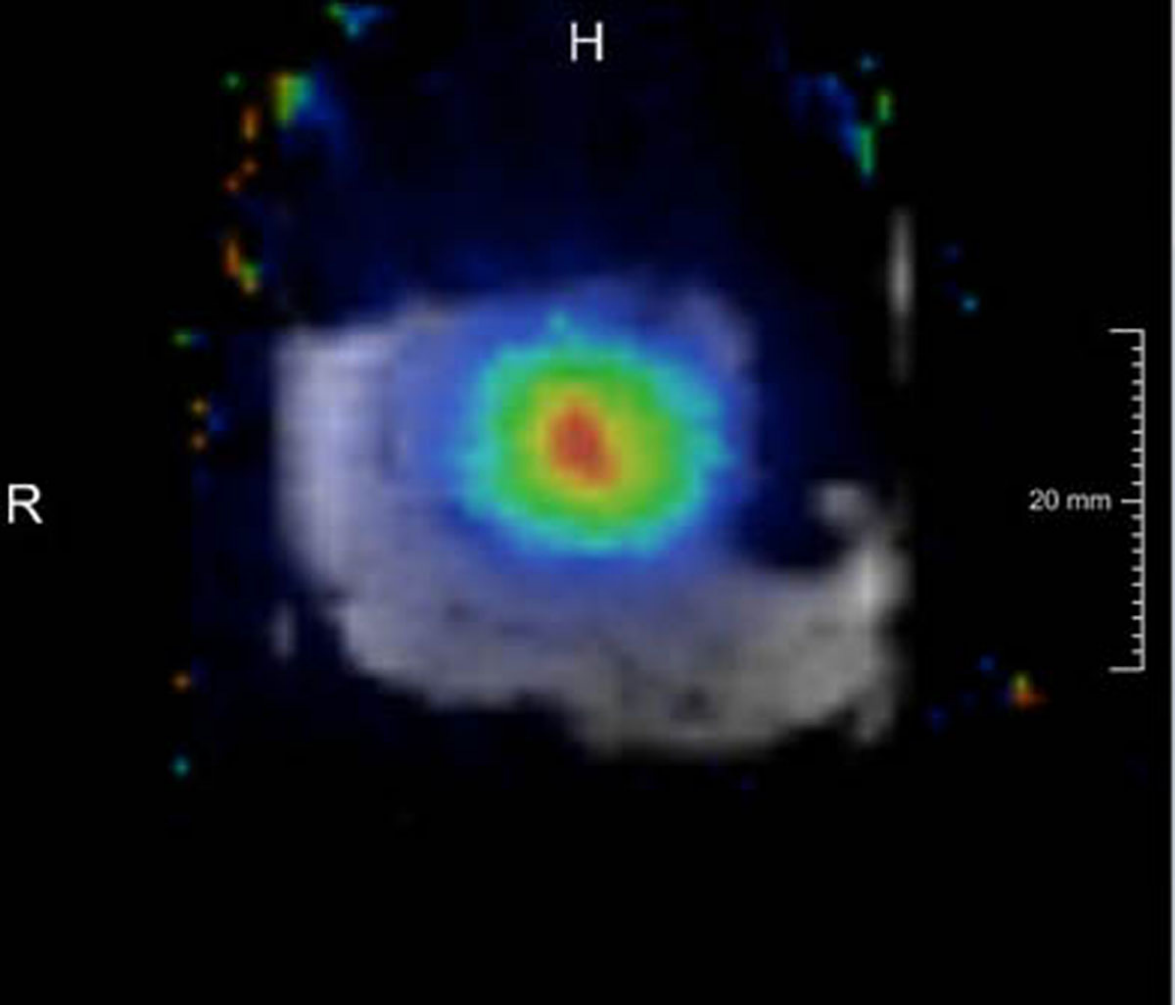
T1w MR image of a meat sample with temperature map overlay.
